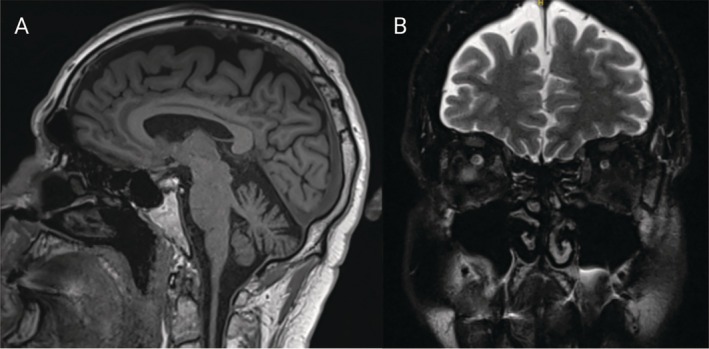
Clinical findings of affected individuals. MRI images of patient A:II:1, showing (A) vermian atrophy on the sagittal section and (B) mild bilateral optic nerve atrophy on the coronal section.
